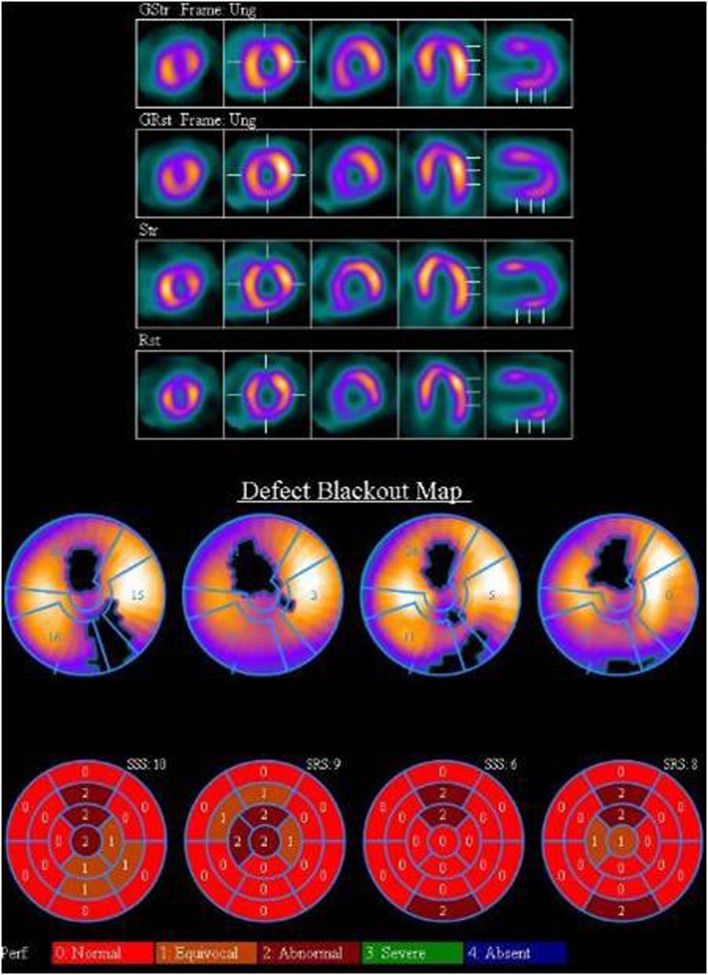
MPI SPECT shows antero-apical, anterior and infero-lateral fixed perfusion defects.
